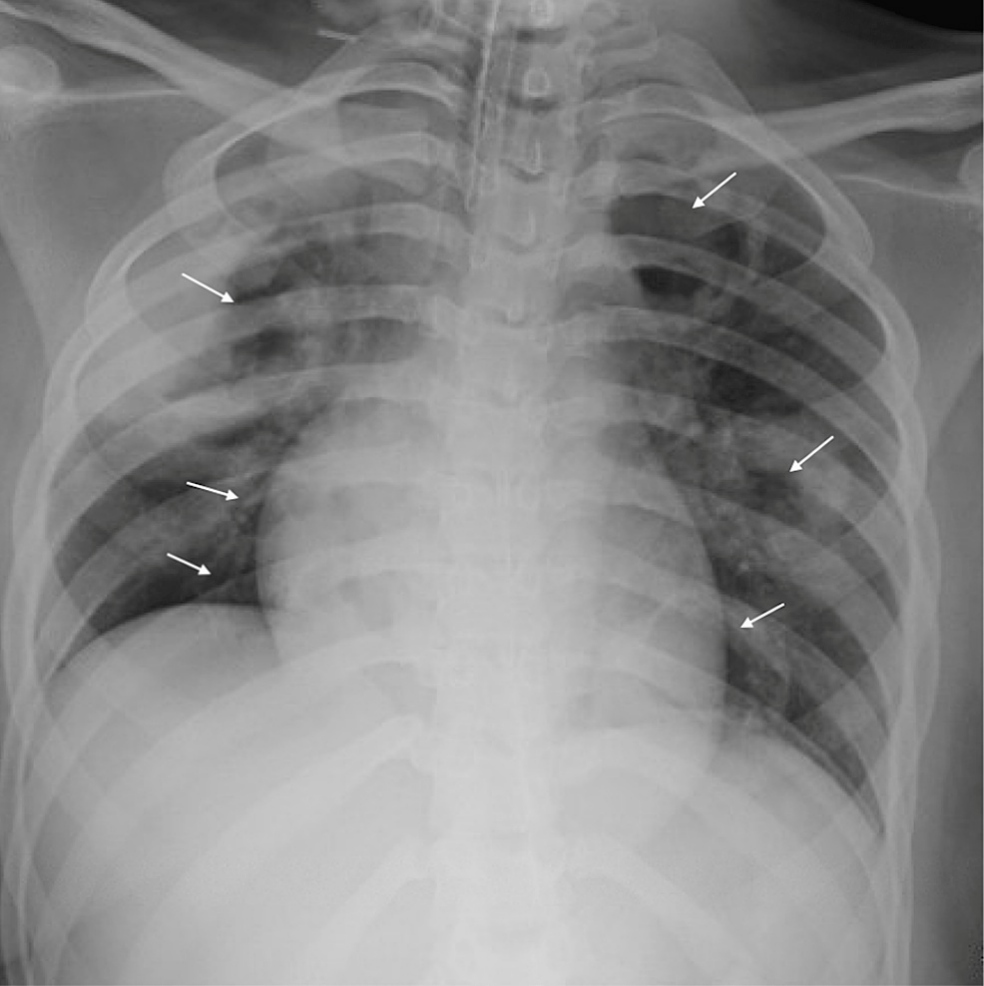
Chest X-ray with granulomatous lesions Arrows point to multiple granulomatous lesions
